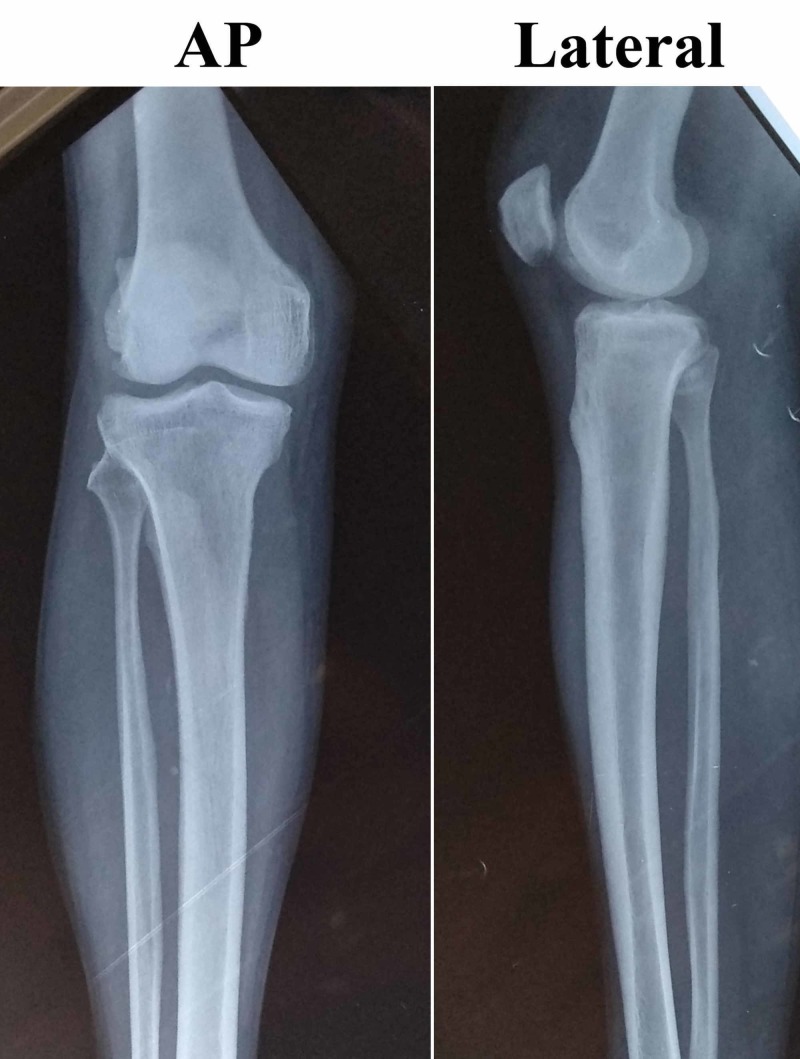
The preoperative X-ray of the right knee joint with proximal leg and distal thigh showing no osteomyelitic changes.
